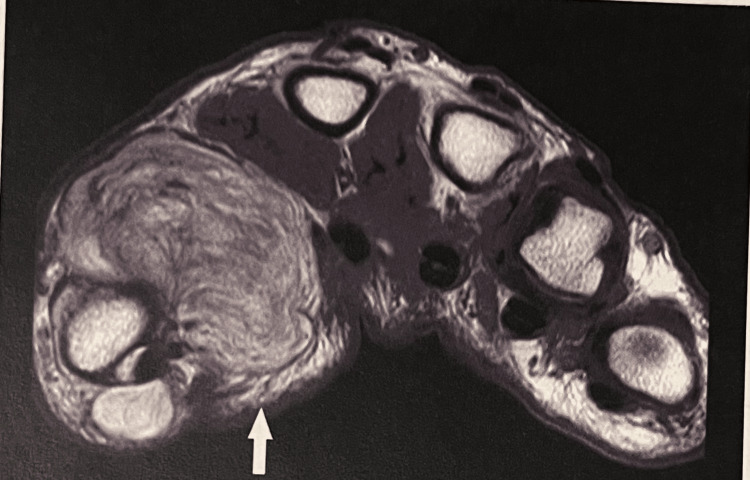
MRI of a first web space lipoma (white arrow).
